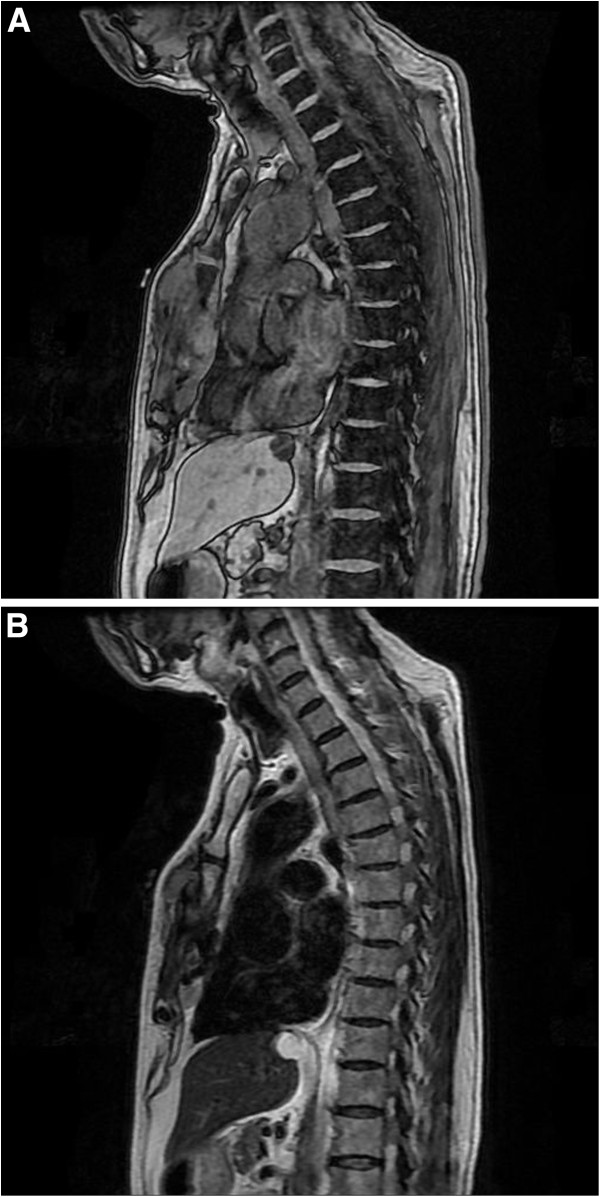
Magnetic resonance T1-weighted image (A) and T2-weighted image (B) of primary site of sternum.
